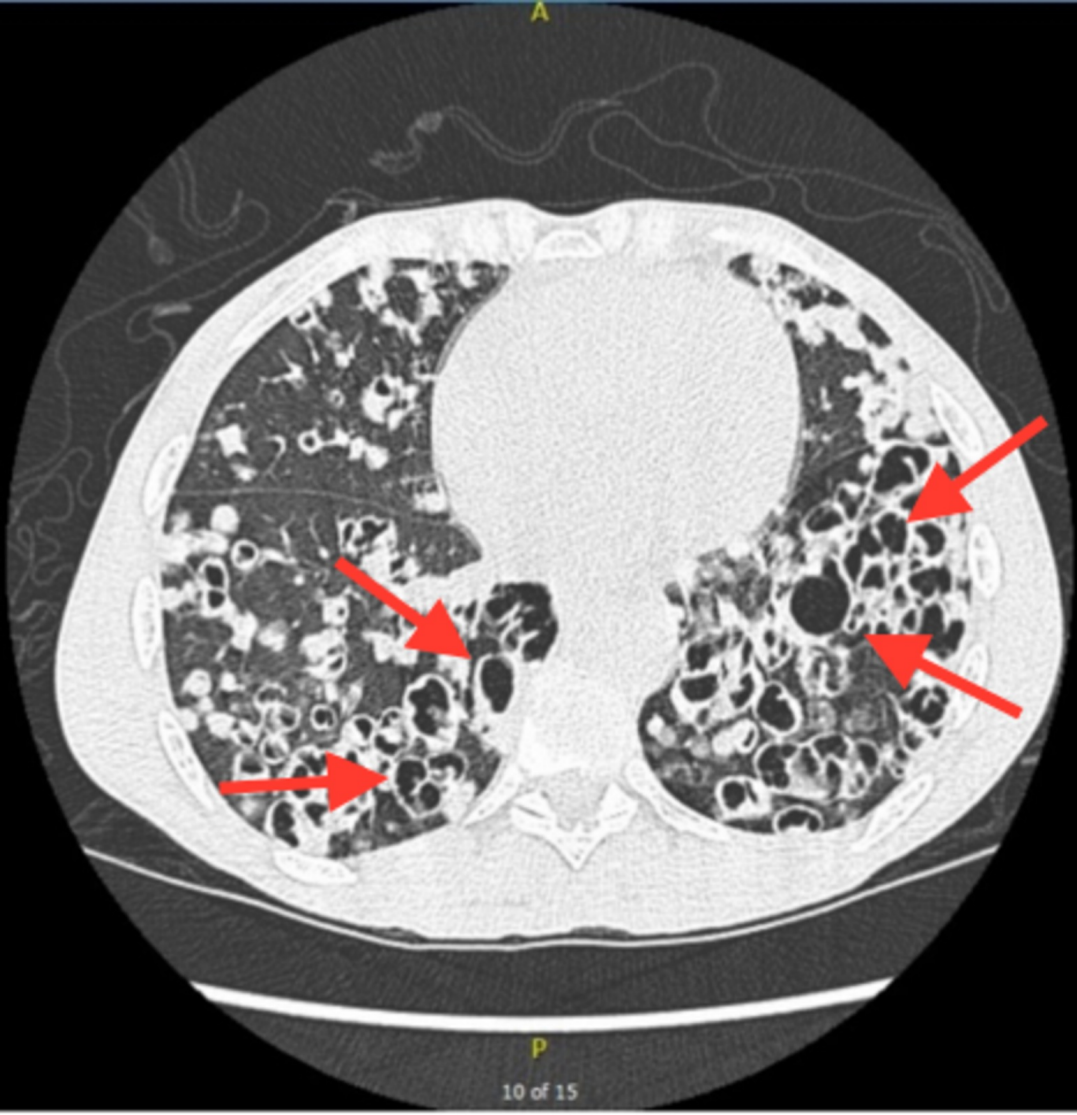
High-resolution computed tomography (CT) revealing multiple thick-walled cysts and nodules throughout bilateral lung fields
